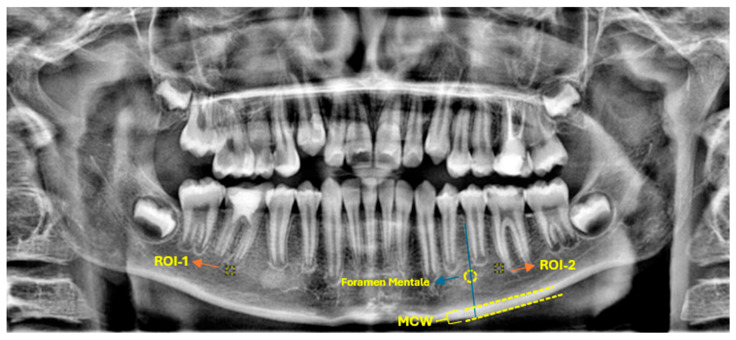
Representative panoramic radiograph demonstrating the selection of ROIs and the methodology for radiomorphometric measurements.
